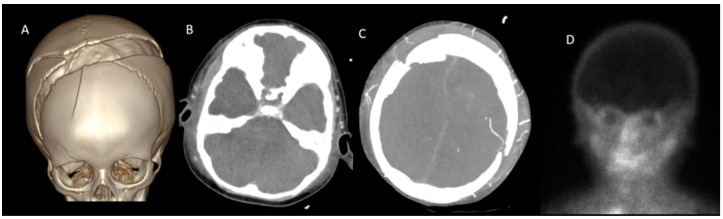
CTA in a 2-year-old boy with clinically confirmed brain death. (A), A cranial fracture is visualized in VR images. (B), A lack of opacification is observed in the SPV and ICV during the venous phase. (C), However, persistent opacification of the right MCA-M4 segment is noted (white arrow). (D), Brain perfusion was absent on scintigraphy, further supporting the diagnosis of brain death. CTA: CT angiography, MCA: Middle cerebral artery, ICV: Internal cerebral vein, SPV: Superior petrosal vein, VR: 3D volume-rendered.
